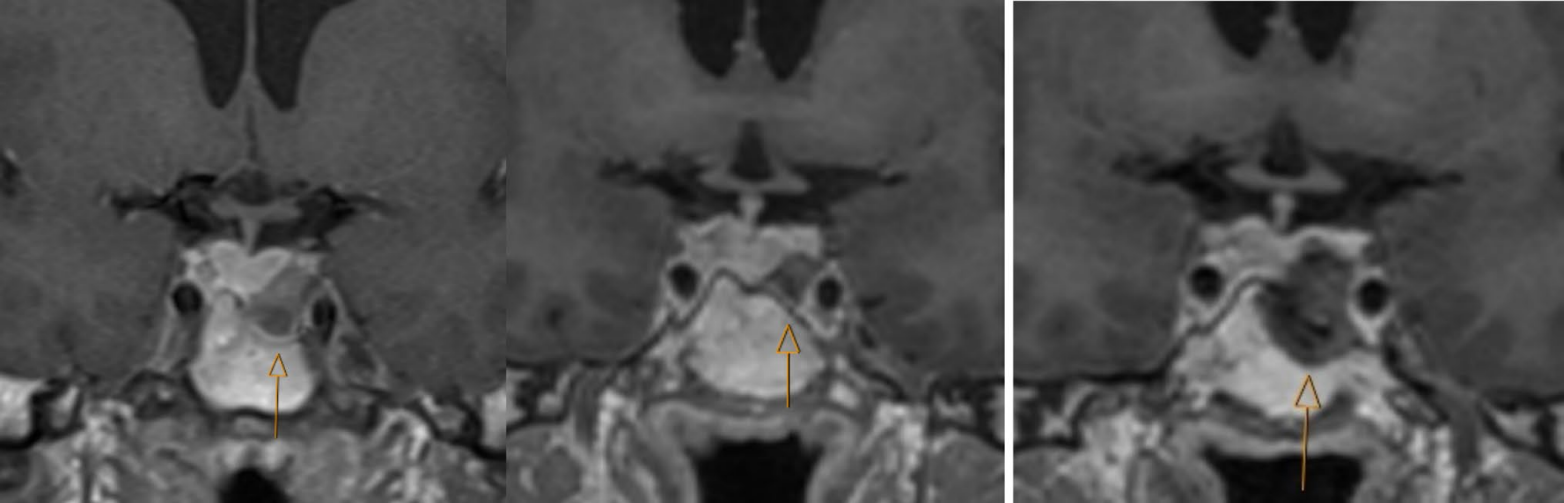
T1 weighted images enhanced with gadolinium. A patient with a macroadenoma at baseline (image to left, arrow) underwent primary surgery in 2015. He was not in remission and medical therapy was indicated. A residual adenoma was visualized (image in the middle, arrow) and through an MDT meeting and shared decision making, the patient underwent repeat surgery in 2023. The MR image to the right is one day after repeat surgery showing postoperative changes (arrow) with no visible residual adenoma. He was in remission after surgery, including at the last visit in 2024
